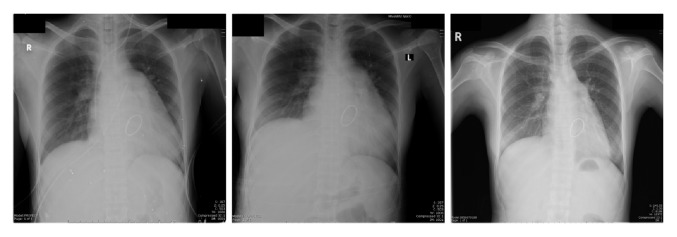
Serial of postoperative chest X-rays showed gradual reduction in cardiac size.
